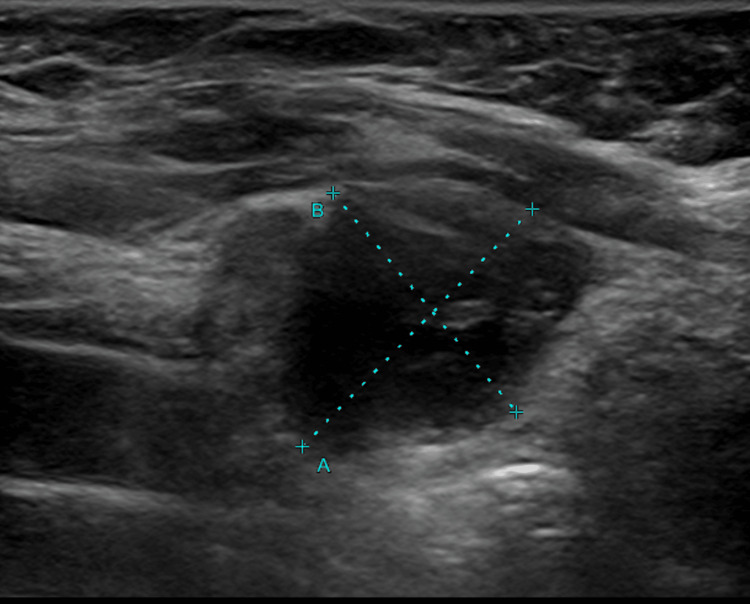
US thyroid showing interval appearance of the left thyroid nodule area marked with blue dotted lines corresponding to a hypermetabolic lesion on comparison PET CT CT: computed tomography; PET: positron emission tomography; US: ultrasound
